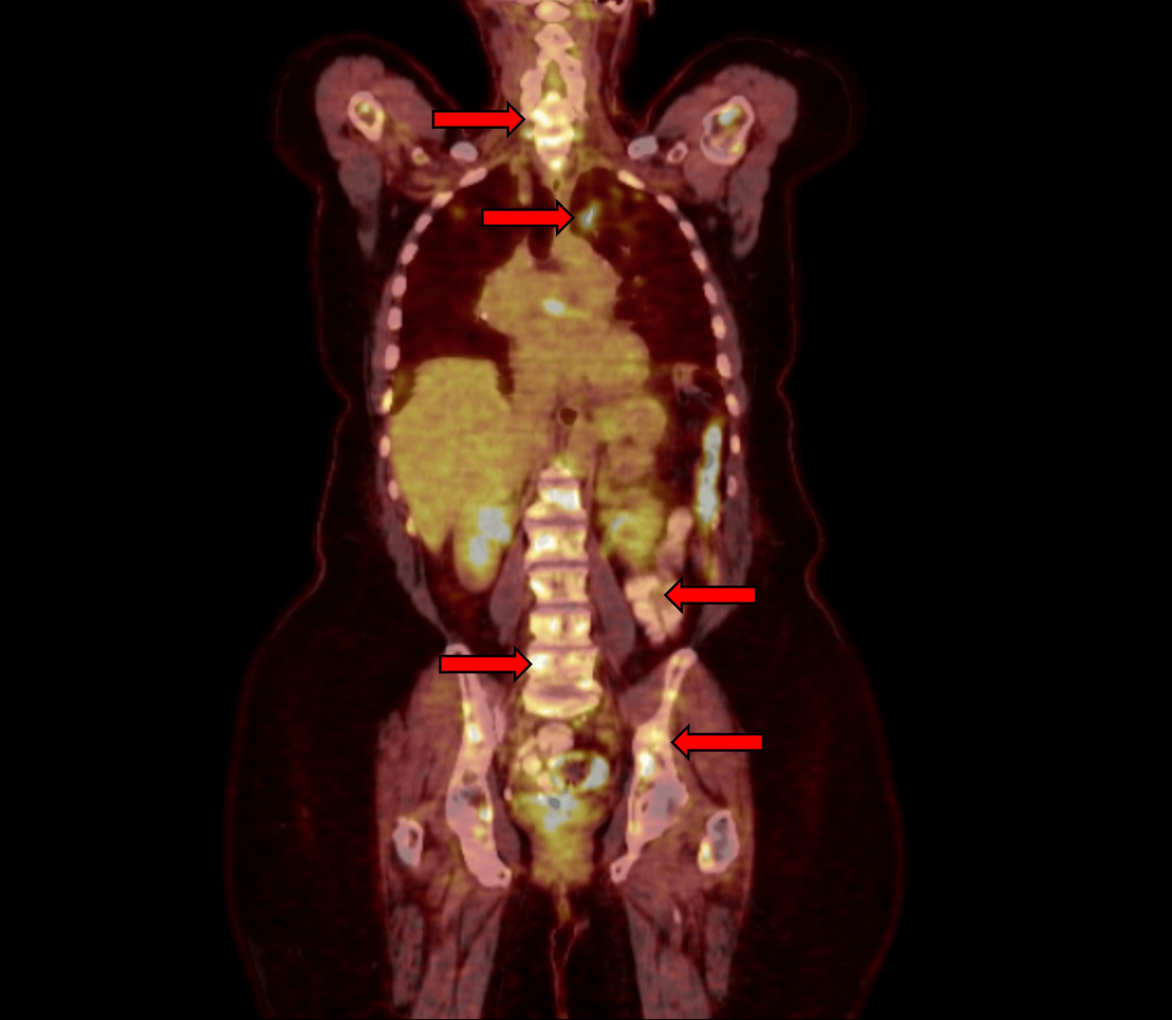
PET-CT scan showing FDG-avid lesions in the bones consistent with osseous sarcoidosis, along with non-osseous FDG-avid lesions in the neck, chest, abdomen, and pelvis FDG, fluorodeoxyglucose
